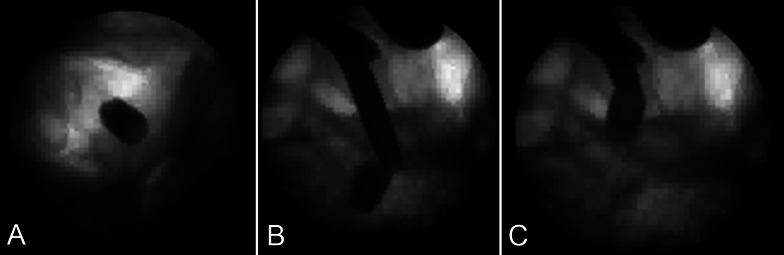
The dental X-ray fluoroscopic equipment DreamRay 60F® (DreamRay, Pusan, Rep. of Korea) was used to identify the location of the displaced implant in a severely superior and posterior location of the sinus. Grasping and suction were used with the “naked eye” to approach and remove the implants in Case 1 (A–C)
